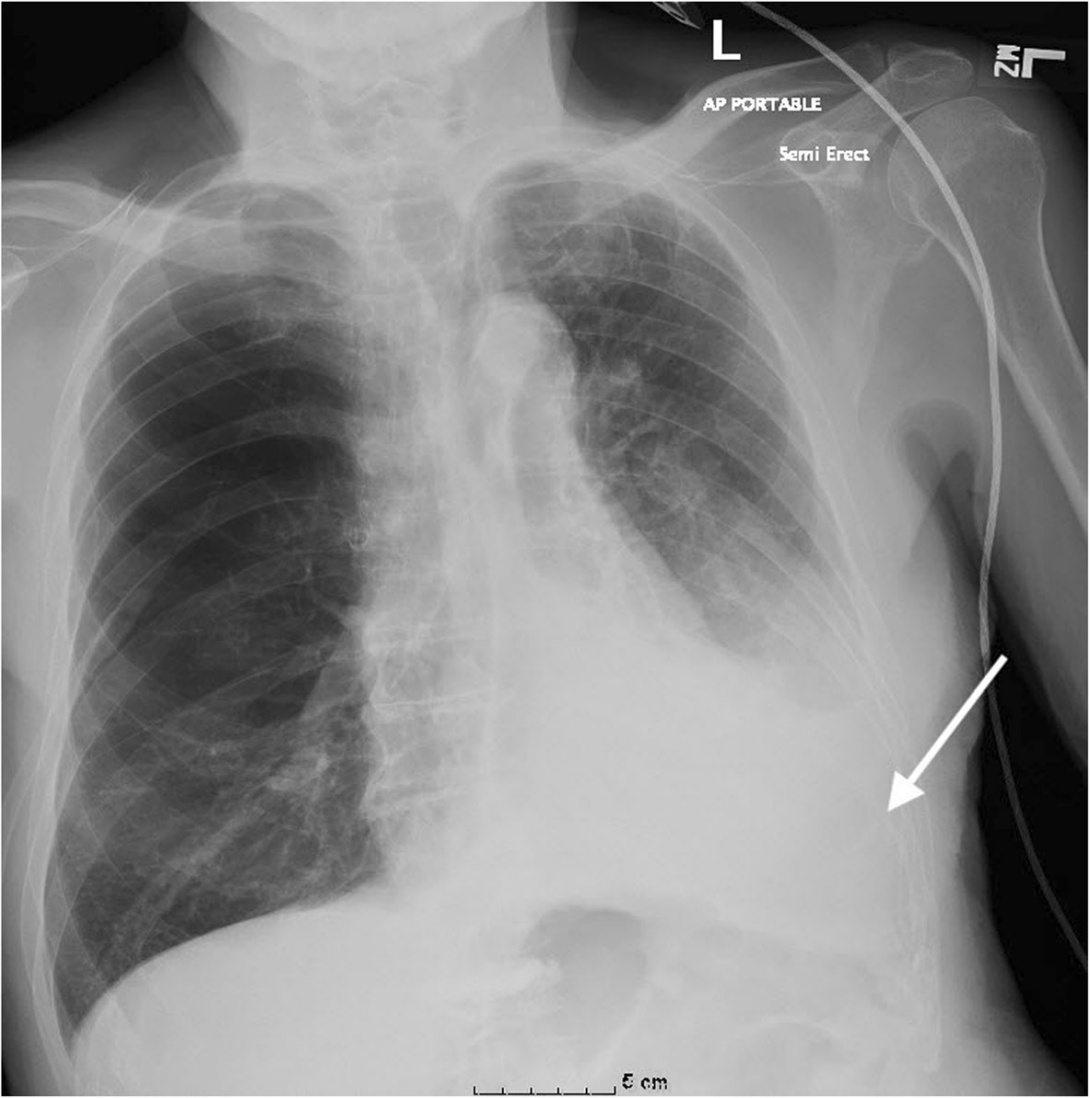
AP (anteroposterior) chest plain radiograph in an 82-year old male, following thoracentesis. Note the significant pleural effusion with compressive atelectasis (arrow) in the left lower hemithorax
